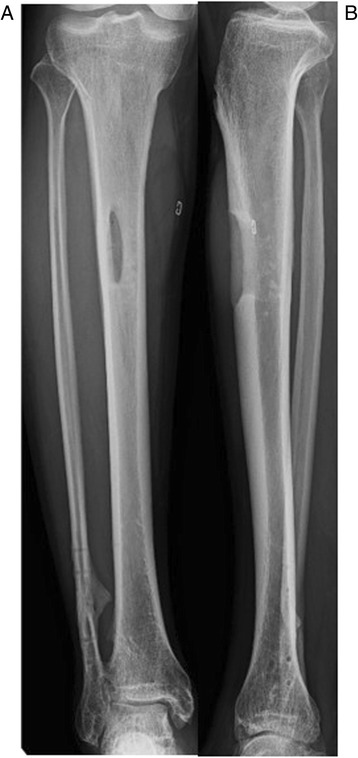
Anteroposterior (a) and lateral (b) radiography of the *right lower leg*. There is no recurrence of osteomyelitis 2 years postoperatively
